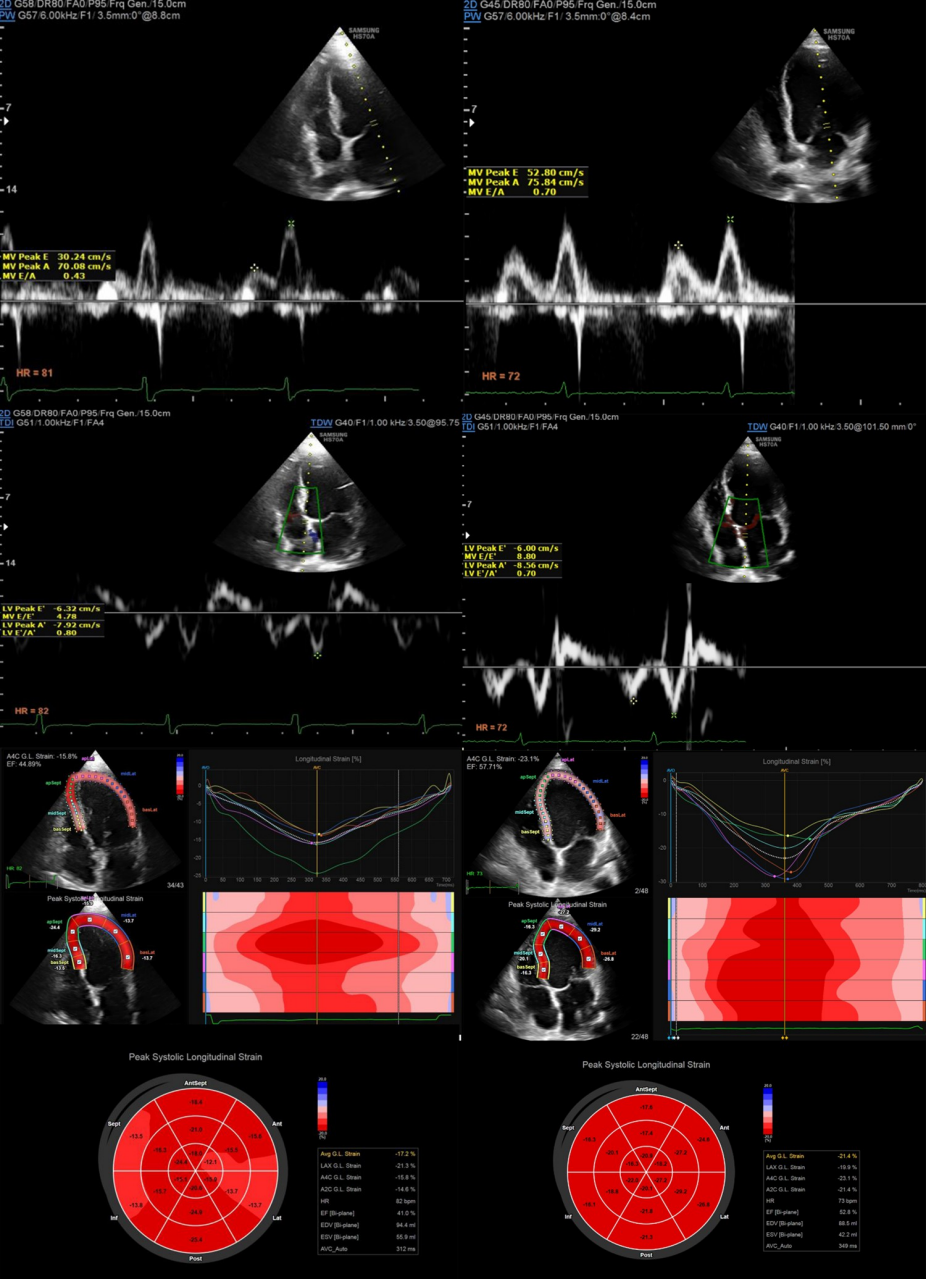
Displays doppler and strain echocardiographic images of patients with DFU before treatment on the left side and after treatment on the right side in order from top to bottom. Firstly, there is a pulsed wave Doppler recording at the miral inflow E and A velocities. secondly, tissue Doppler imaging at the medial mitral annulus shows e’ and a’ velocities. Thirdly, a region of interest tracing in the apical 4-chamber views shows segments of left ventricle and peak systolic strain waveform in mid-panel. Fourthly, bull’s eye plot of longitudinal strain is shown, diplaying strain values of each segment.
